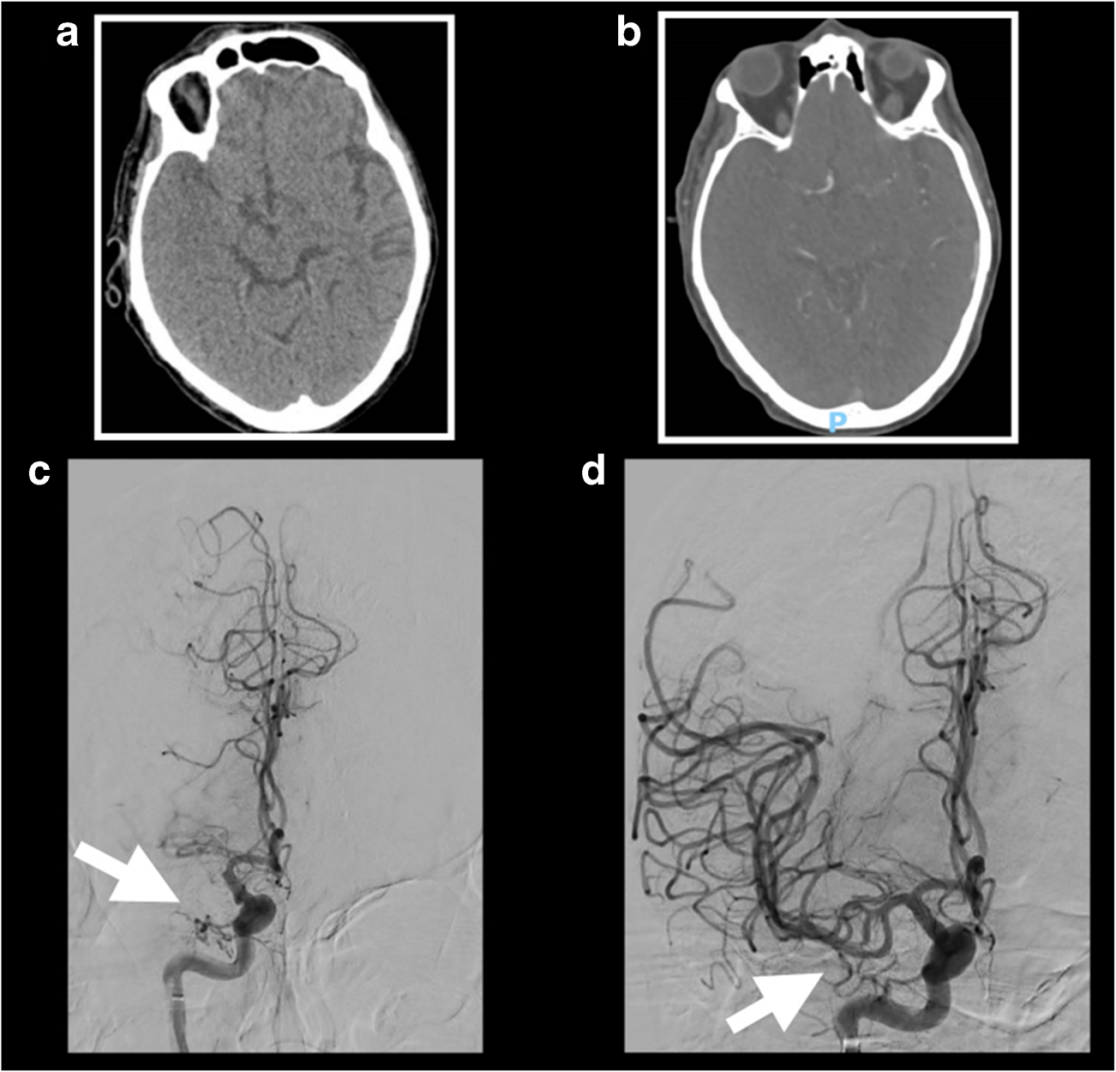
Case 2. a Axial CT head showing hyperdensity of the M1 segment of the right middle cerebral artery consistent with acute thrombus, and focal hypodensity of the right temporal lobe with loss of grey-white matter differentiation. b CT angiography demonstrating abrupt cutoff at the proximal M1 segment of the right middle cerebral artery. c Angiography confirmed occlusion of the right middle cerebral artery. d Post-angiographic images demonstrating the restoration of the flow in the right middle cerebral artery following successful thrombectomy
